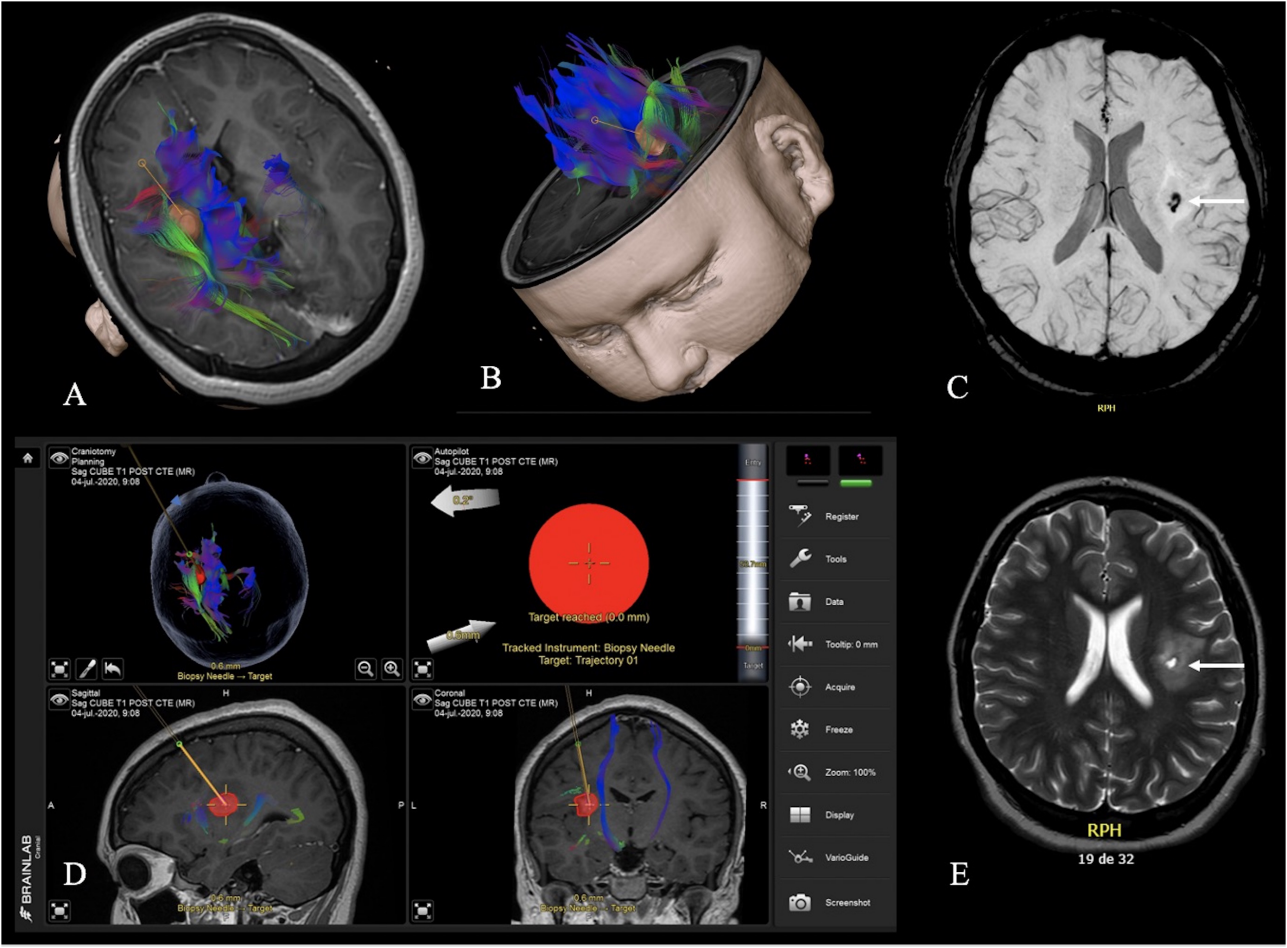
3D reconstruction showing a left insular tumor, its relationship with the motor bundle and the arcuate fasciculus, and the biopsy trajectory (A, B). Screenshot of the planned biopsy tract to the target (D). Intraoperative MRI with a T2-weighted (E) and SWI (C) as a final control to verify the location of the tumor samples indicated by the arrows and to rule out complications related to the procedure.
